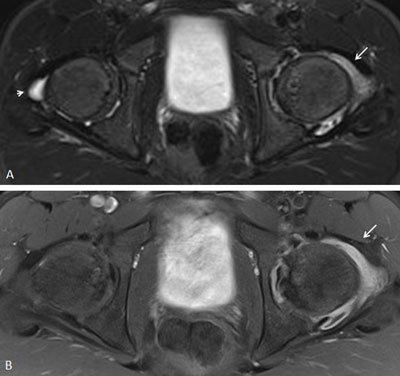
Hip arthritis in a 14-year-old boy with JSpA. a. Axial STIR image shows a joint effusion in the left hip joint (arrow). Note also the small amount of fluid in the right hip joint. b. Axial contrast-enhanced fat-saturated T1-weighted image shows synovial enhancement at the left hip joint (arrow). No enhancement is seen at the right hip joint.
